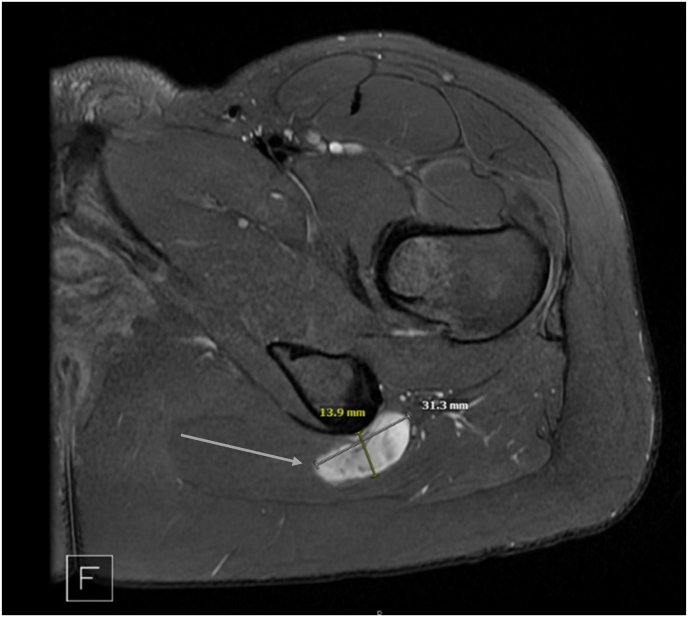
Lumbar Spine MRI. Lumbar Spine MRI revealing 3.1 cm nerve sheath tumor in the ischiogluteal space at the level of the inferior gemellus with mass effect on the sciatic and inferior gluteal nerves as indicated by the arrow.
